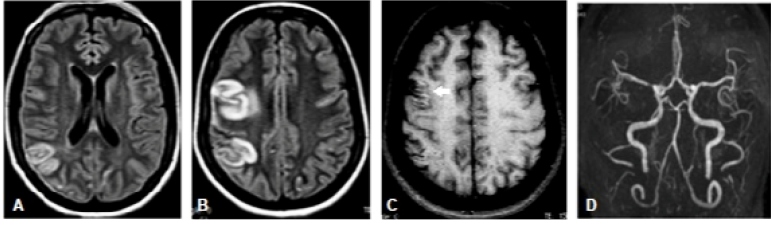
MRI. Axial T2 FLAIR weighted images (A, B). T1-weighted axial images without venous contrast (C) and arterial 3D TOF MRA (D). We noticed hypersignal on T2/FLAIR at the right frontoparietal lobes , with spinning thickening and mild vasogenic edema, corresponding to ischemic arterial vascular injury. There is also cortical spontaneous hypersignal on T1, without expression on T2* images, related to cortical laminar necrosis. There is no mass or reduction on the ADC map on these changes (not shown). We noticed slight signal loss in the proximal segment (M1) of the right middle cerebral artery, in addition to clear asymmetry of its distal segments (M3), with mild vascular poverty to the right (D). MRI, magnetic resonance imaging; FLAIR, fluid-attenuated inversion recovery; MRA, magnetic resonance angiography; 3D TOF, three-dimensional *time of flight;*
